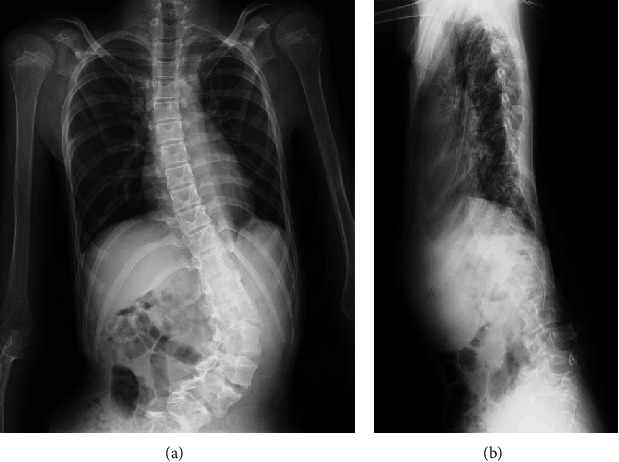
(a) Preoperative X-ray—anterior-posterior (AP) view of levoscoliosis (T10–L5: 80°) and (b) preoperative X-ray—lateral view.
